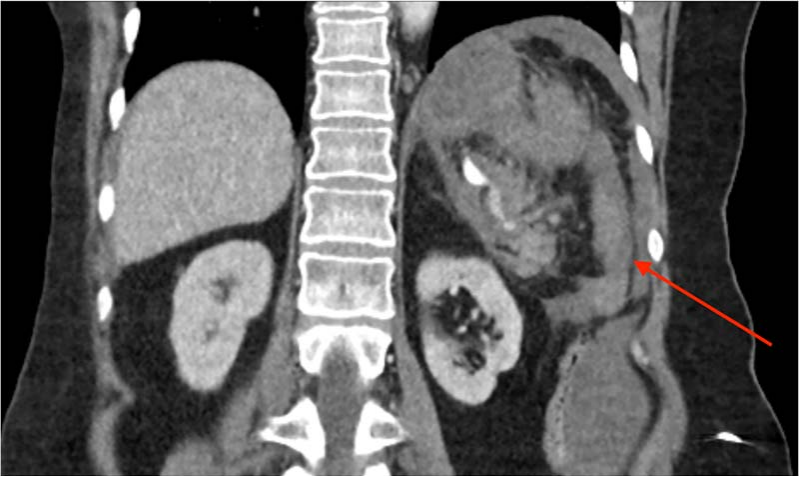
CT of the abdomen and pelvis, coronal plane, arterial phase. Large intraabdominal haematoma in the left upper quadrant.
